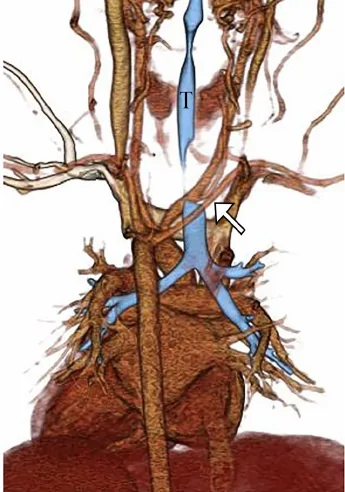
Three-dimensional computed tomography image showing tracheal stenosis (T) and an aberrant right subclavian artery traversing dorsally (arrow).
